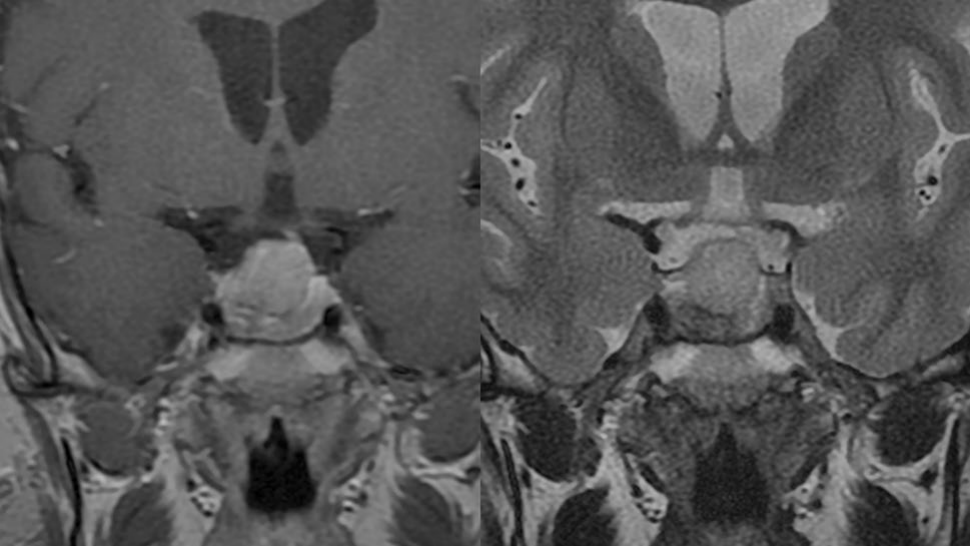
Case no. 7, coronal T1 weighted after Gd-DTPA (left image) and T2 weighted (right image) MR imaging revealed invasive pituitary macroadenoma invading sellar floor
